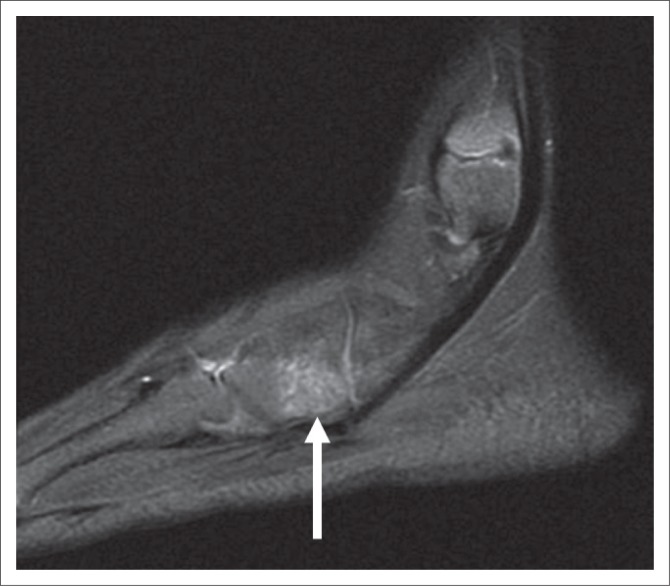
Cuboid contusion. Sagittal fast spin echo T2-weighted image (Repetition Time [TR]/Echo Time [TE] 3500/73 ms) of the lateral aspect of the foot shows focal oedema-like signal within the cuboid. No fracture line was evident. The finding presumably accounted for the patient’s lateral foot pain at presentation.
